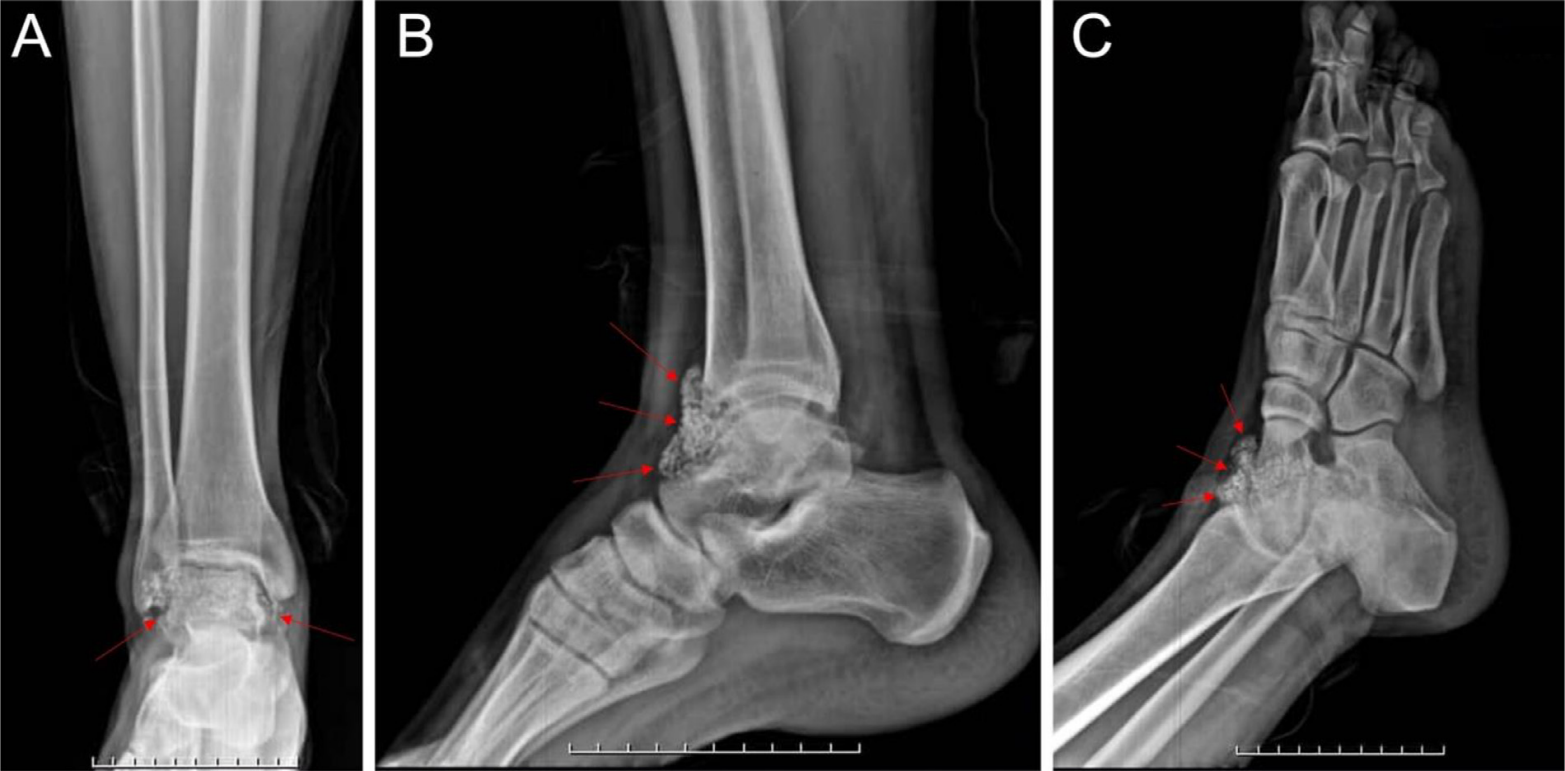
Preoperative plain radiographs of the right foot and ankle showing calcified synovial chondromatosis lesions (loose bodies) in the anterior part of the ankle, indicated by red arrows. Loose bodies, which appear as discrete calcified densities, are seen in A) Anteroposterior (AP) view, (B) Lateral view, and C) Oblique view.
